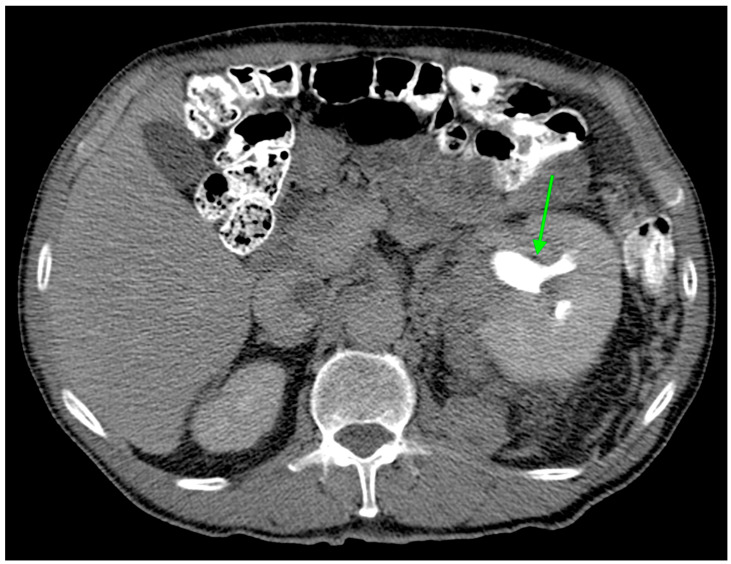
Axial CT image during excretory phase showing ccRCC with AFDP gene expression with hydronephrosis (green arrow).
